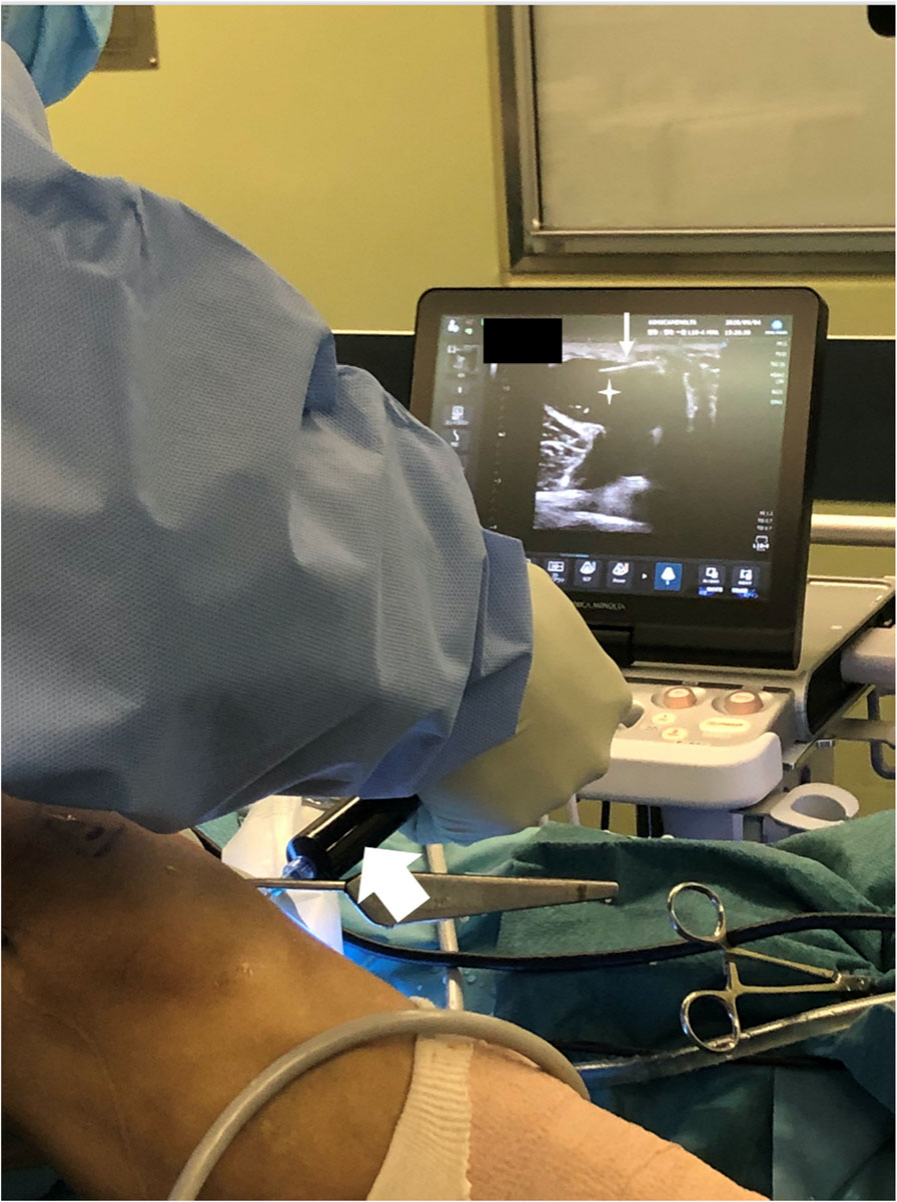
A contrast dye (indigo carmine, broad white arrow) is injected into the popliteal cyst (star) percutaneously using ultrasonography. The 23-gauge needle (narrow white arrow) is clearly visible on the ultrasound screen
